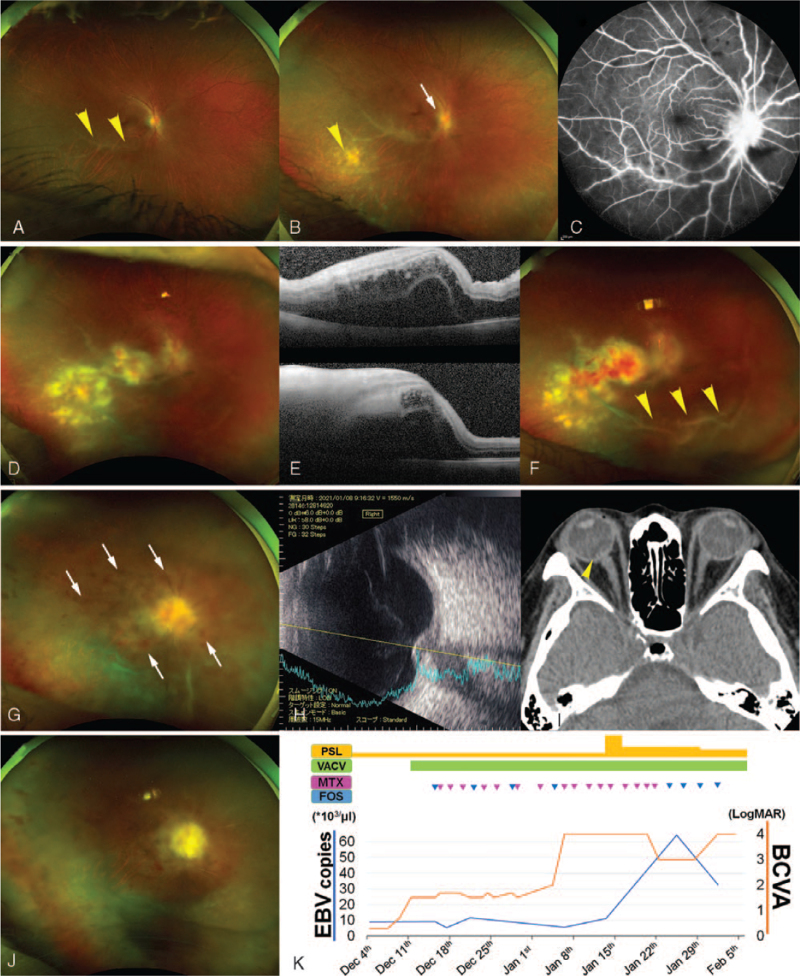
Clinical course of the patient with Epstein–Barr virus intraocular infection. A unilateral exudative change along the vessel appears at the temporal retina of the 44-year-old patient on October 30, 2020 (A, arrowheads). A snowball-like vitreous opacity, recent retinal spot (B, arrowhead), and peripapillary exudative changes (B, arrow) have developed by November 26, 2020 (B), detected on fluorescein angiography (C). By December 11, 2020, the retinal lesions, such as retinal infiltration and hemorrhage, have progressed to involve the macula (D), with severe edema and exudative detachment observed on optical coherence tomography (E, upper, horizontal image; lower, vertical image). Retinal hemorrhage and vitreous opacity have been observed on December 14, 2020 (F, arrowheads). On January 6, 2021, she has developed central retinal vein occlusion (G, hemorrhage is pointed by arrows) related to the swelling of the optic-nerve papillae recorded, using echography (H) and computed tomography (I, arrowhead). However, the vitreous infiltration is suppressed (G). The activity of the optic-nerve lesion and the retinitis and vitreous infiltration are suppressed on January 28, 2021 (J). (K) depicts the EBV copy numbers in the aqueous humor and best-corrected visual acuity over time, in relation to the administered drugs. Each triangle denotes the intravitreal injection (MTX, pink; foscarnet, blue). EBV = Epstein–Barr virus, FOS = foscarnet, MTX = methotrexate, VACV = valaciclovir hydrochloride.
